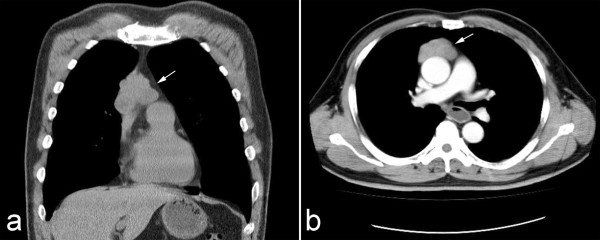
Preoperative chest radiology of lesion. (a) Chest CT image showed a well-defined mass in right anterior mediastinum (white arrow). The tumor was observed to adhere to the wall of great vessel. (b) The contrast enhanced CT scan showed the solid mass with mild heterogeneous enhancement adhered, but not invaded the wall of great vessel.
